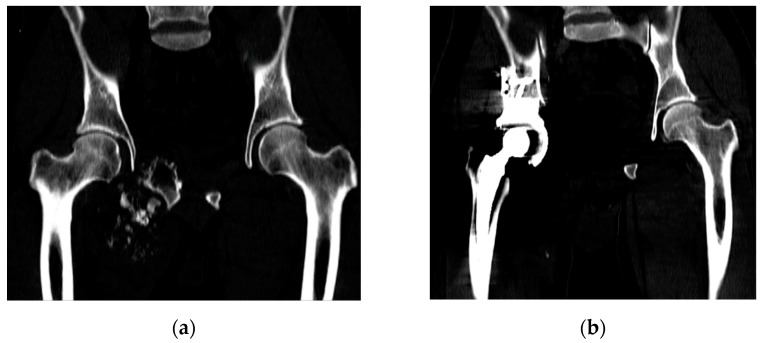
CT images: (a) before surgery; (b) after surgery.
